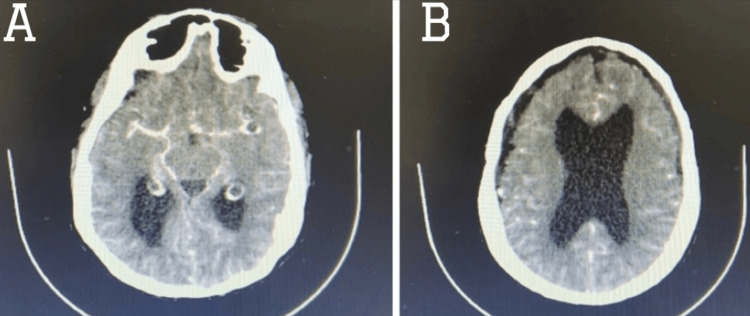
Axial cut of the CT angiogram of the brain Axial cut of the CT angiogram of the brain showed no vascular malformation in (A) the areas surrounding the circle of Willis and (B) the cortical frontoparietal areas.
